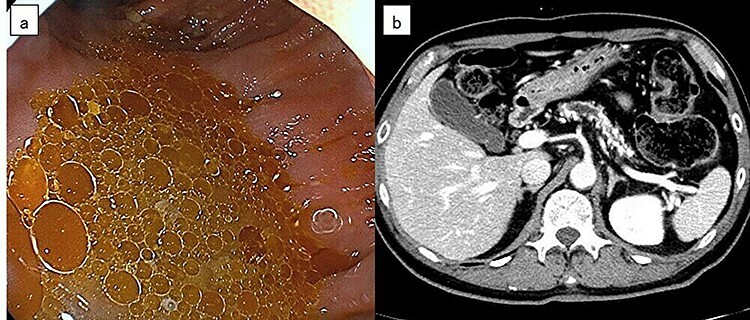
(a) Lower gastrointestinal endoscopy revealed a large amount of oil droplets floating in the large intestine. (b) Abdominal computed tomography showed numerous pancreatic stones in the pancreas and the main pancreatic duct was irregularly dilated. The patient was diagnosed with chronic pancreatitis.
